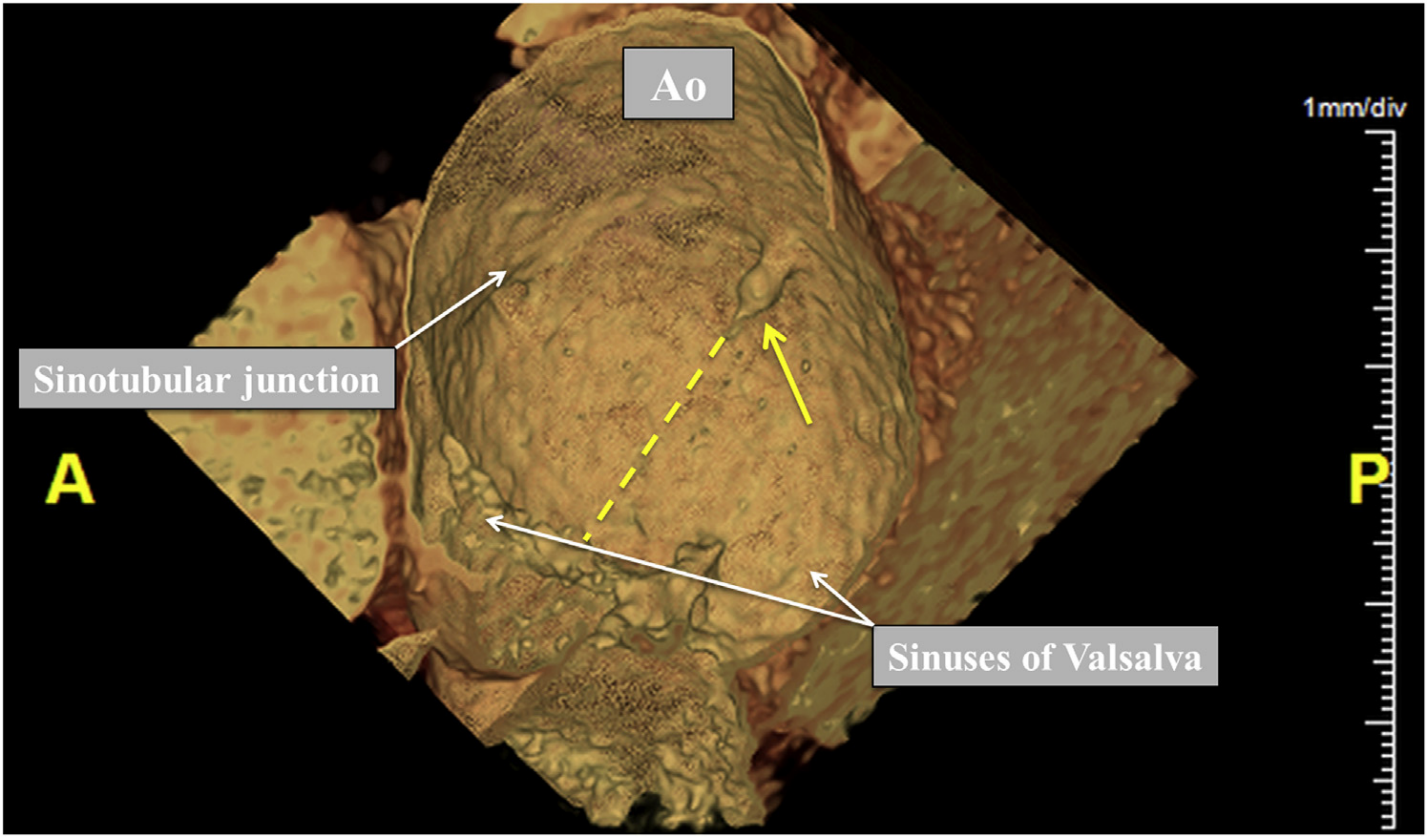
Three-dimensional CT scan showing the remnant of a fibrous strand (*yellow arrow*) and its former trajectory toward the aortic valve (*yellow dotted line*). *Ao*, Aorta.
